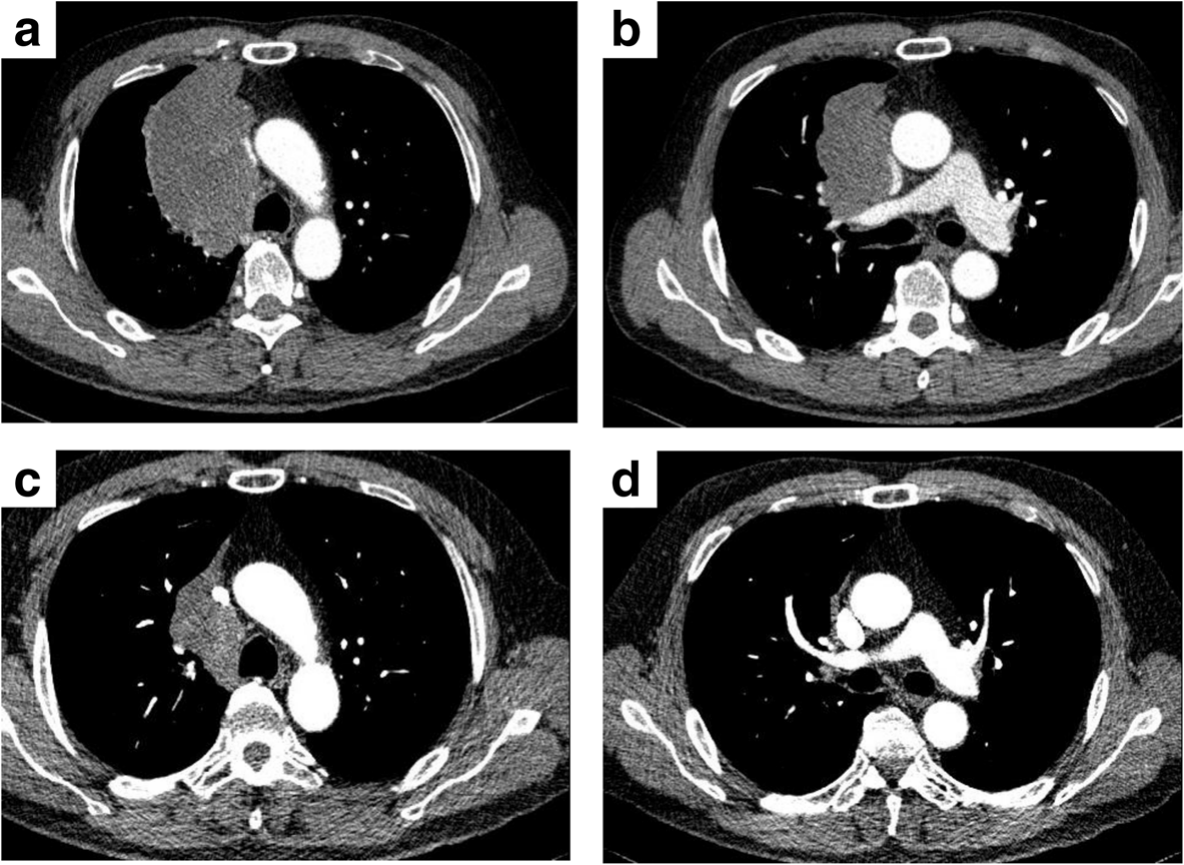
a, b Chest computed tomography (CT) before treatment showed an 11.0-cm mass in the right upper lobe that had invaded the superior vena cava (from the right internal jugular vein to the right atrium). c, d CT after chemotherapy showed a 5.6-cm mass in the right upper lobe. The reduction rate of the tumor size was 49.1%
